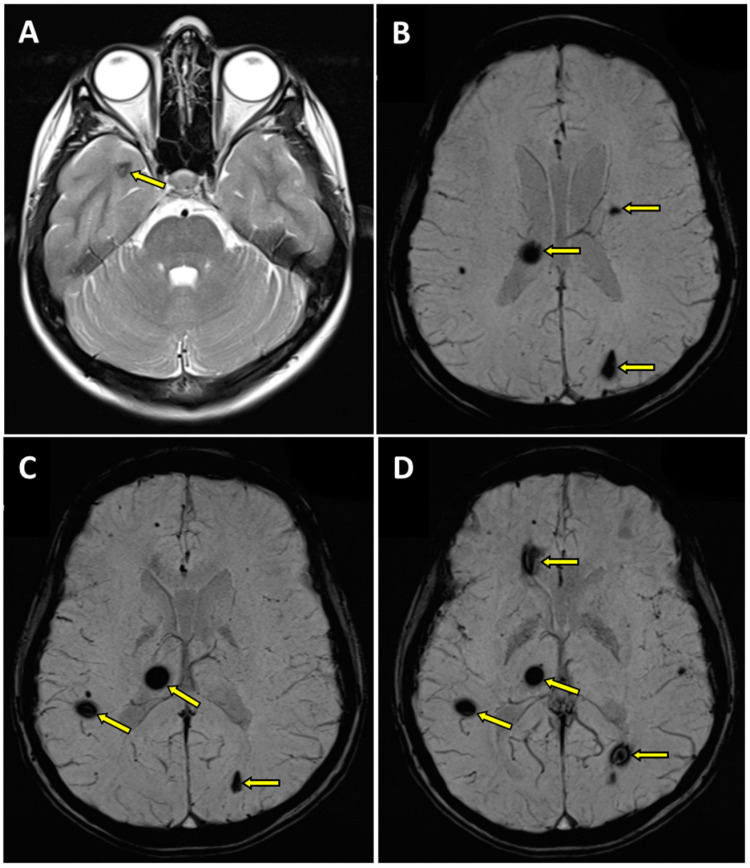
Magnetic resonance imaging (MRI) of the brain in T2 (A) and SWI (B–D) sequences. Transverse projection MRI showed the presence of cavernous hemangiomas within the right thalamus with hemosiderin deposits (B–D), at the fronto-parietal junction on the right side (C,D), in the semioval center on the left side (B), in the left occipital lobe (B–D), in the right temporal lobe (A), and in the right frontal lobe (D); pathologic changes marked with an arrow.
